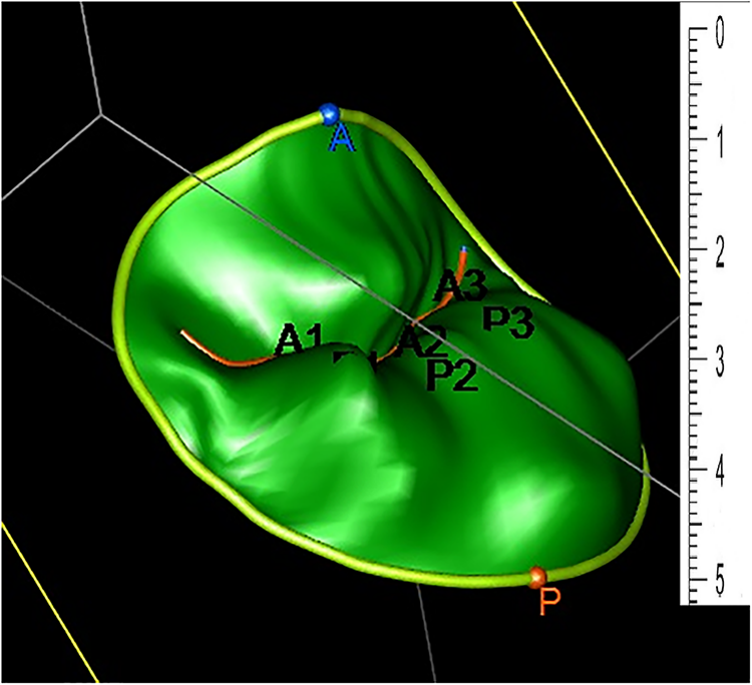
Three-dimensional geometrical Model generated based on an echocardiographic image. The two leaflets are divided into a total of six scallops: A1, A2, A3 (anterior) and P1, P2, P3 (posterior).
